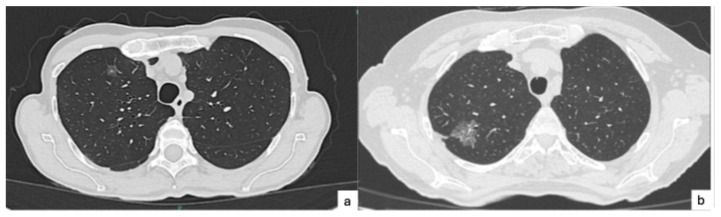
Two cases of STAS-negative lung cancers, as they appeared on CT scan. (a). Right upper lobe lung lesion presenting as ground-glass opacity with central solid component undergoing segmentectomy, later revealing to be invasive lung adenocarcinoma pT1a pN0, STAS-negative. (b). Right upper lobe lung lesion presenting as ground-glass opacity with central solid component undergoing lobectomy, later revealing to be invasive lung adenocarcinoma pT2a pN0, STAS-negative.
